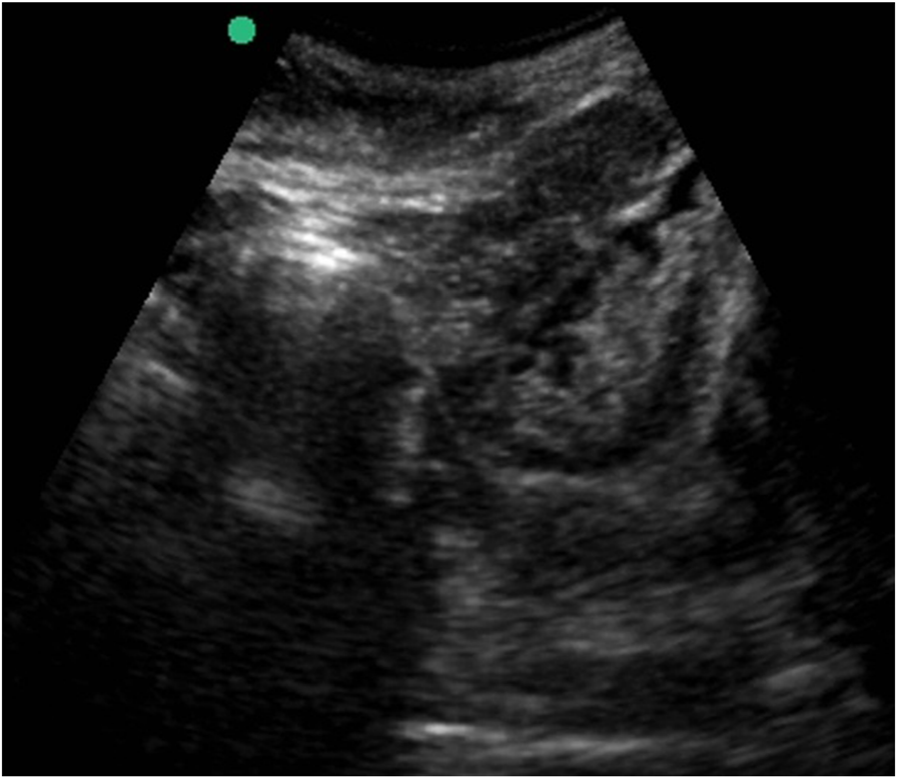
Ultrasound localisation of cadaveric kidney
